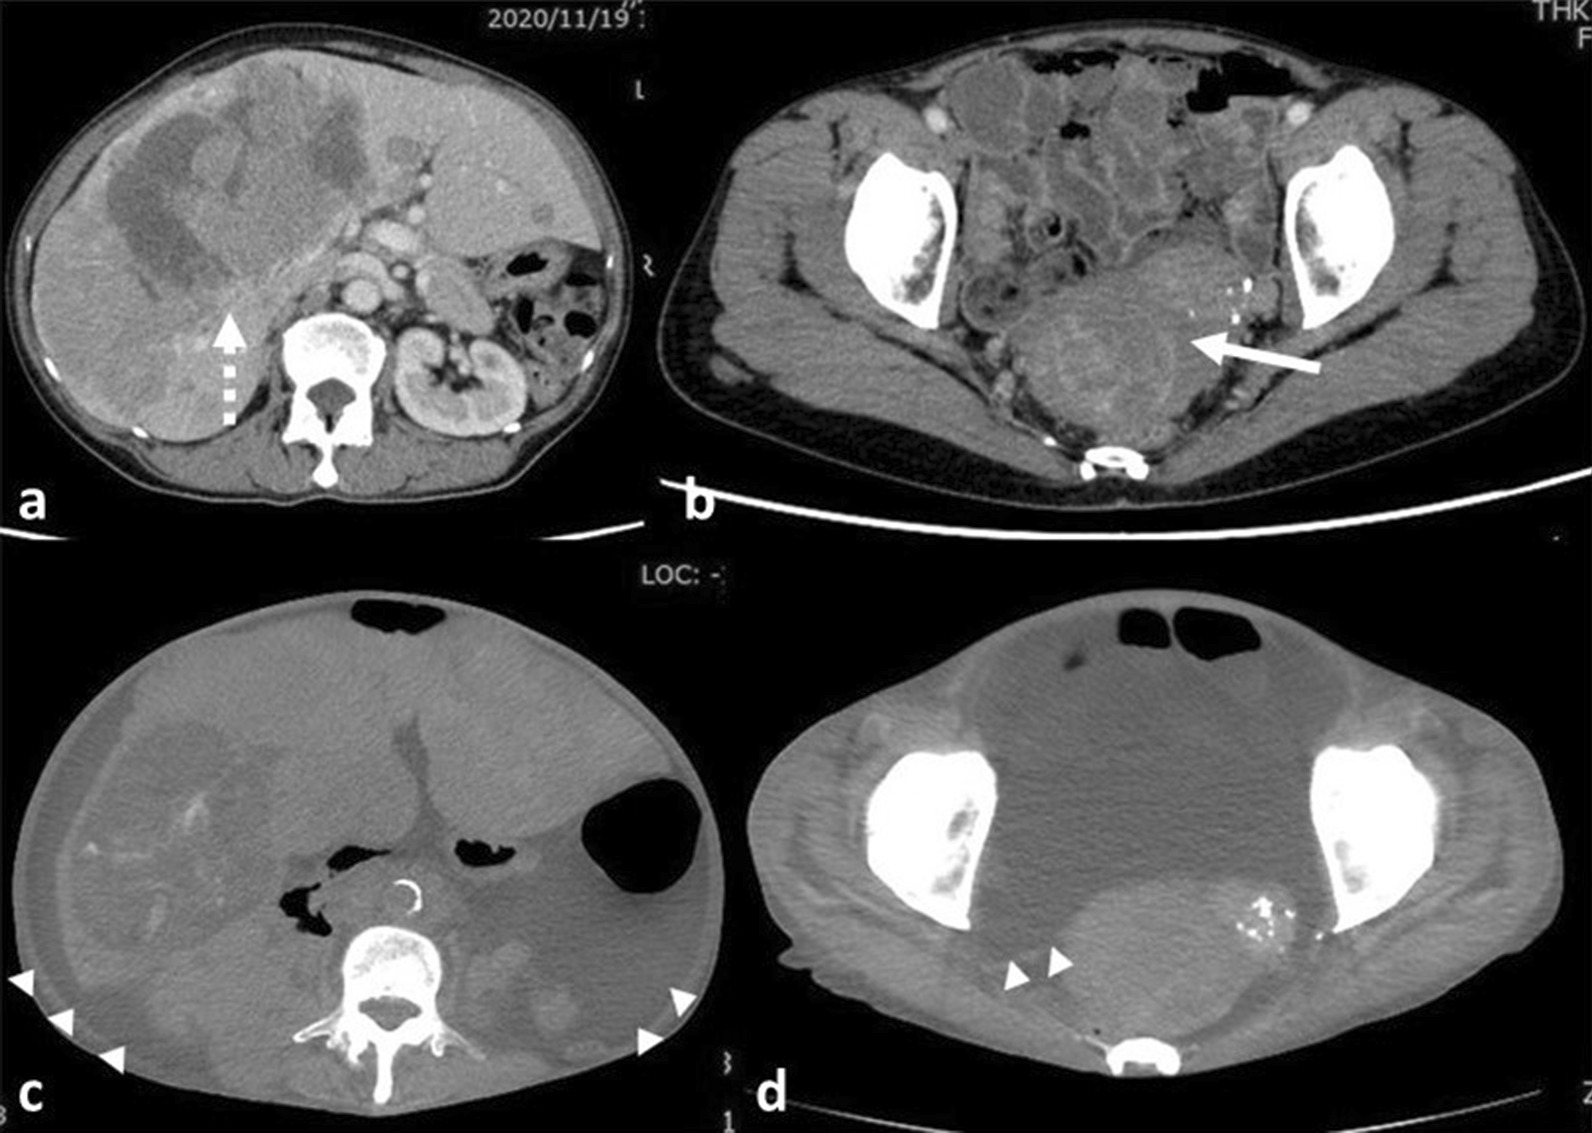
Computed tomography scans. a, b Enhanced body computed tomography scan taken in November 2020, showing a lesion 7 × 8 cm^2^ in size in the cavity of the lesser pelvis (solid arrow), as well as multiple liver metastases (dotted arrow). c, d Plain body computed tomography scan taken on 23 May 2022 (final hospitalization). The largest liver metastasis showed decreasing size, but ascites were present (arrowheads), and there were also findings of possible additional bone metastases (not shown)
